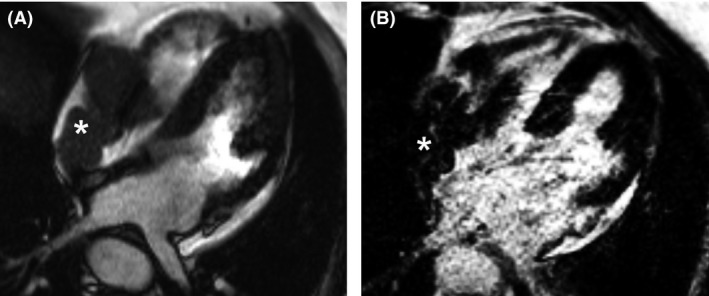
Cardiac MRI (four‐chamber view) from patient B. (A) Still frame from cine series showing the tumor (*) expanding from the right atrium toward the tricuspid valve and the right ventricle, and partly obstructing the tricuspid valve. (B) Late gadolinium enhancement showing partial washout in the mass (*), consistent with a malignant tumor.
